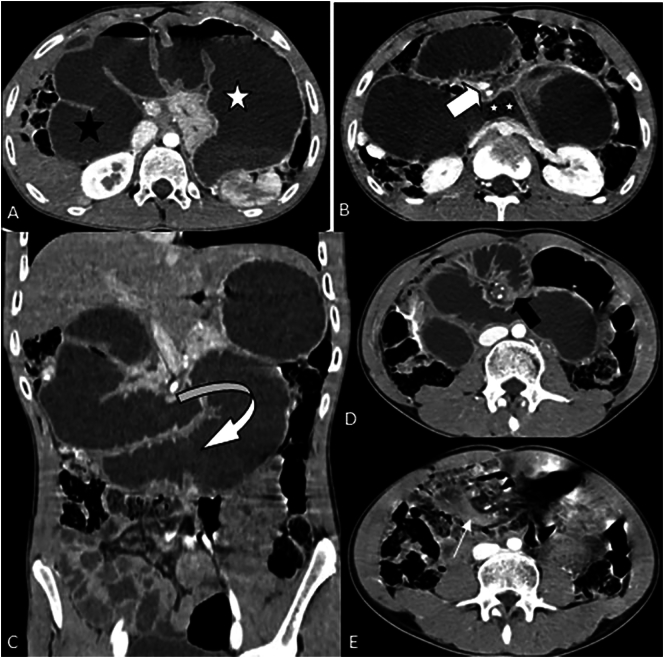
a-e: CT scan slices shows that there was a markedly dilated stomach, duodenum, and jejunum filled with fluid, the largest measuring 6.2 cm. The dilated small bowel abruptly transitions into the mid abdomen with a swirled appearance of mesenteric vessels (SMA and SMV). There was a distally collapsed small bowel to the point of the swirled sign in keeping with mid-gut volvulus. No abnormal wall thickening nor mass lesion.
